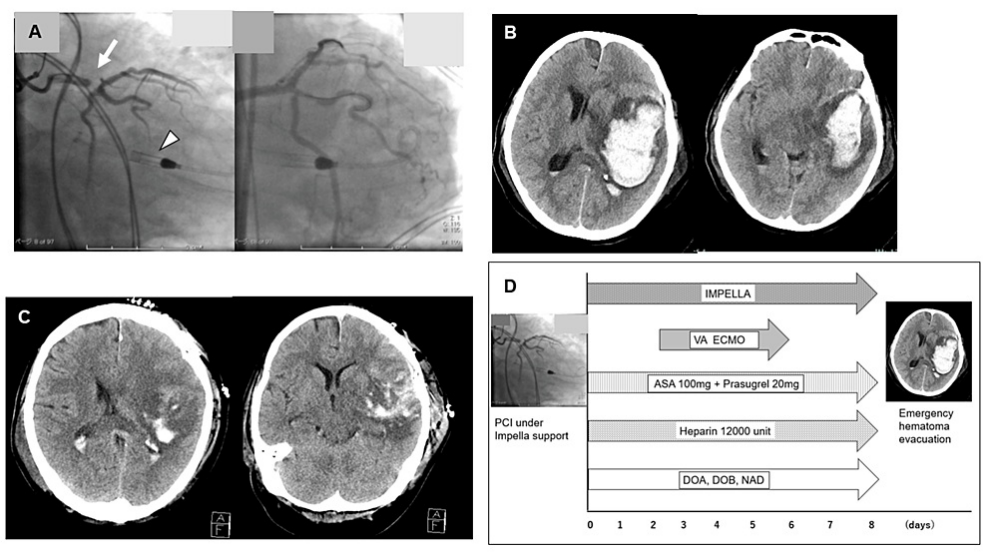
Clinical course of case one A) Images of the left anterior descending artery before (left) and after (right) percutaneous coronary intervention: Severe stenosis of the left anterior descending artery was treated by percutaneous transluminal angioplasty and stent placement. The Impella was implanted to treat cardiogenic shock; B) Intracerebral hemorrhage in the left frontal and temporal lobes. The hematoma volume is 59.3 mL; C) Computed tomography after emergency hematoma evacuation shows that the hematoma had been removed; D) Graph showing the treatment process for case one from admission to the onset of intracerebral hemorrhage VA ECMO - venoarterial extracorporeal membrane oxygenation; PCI - percutaneous coronary intervention; ASA - acetylsalicylic acid
